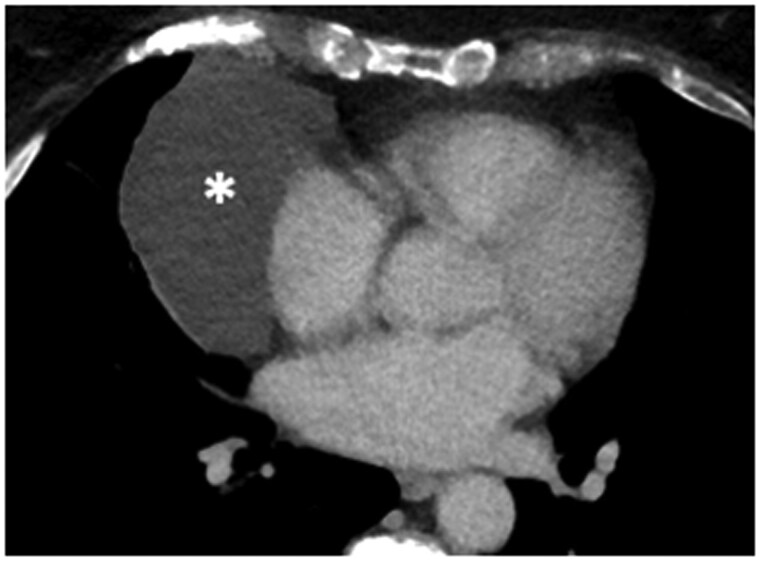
Pericardial cyst. Contrast-enhanced axial CT image of the chest reveals a lesion (asterisk) in the right cardiophrenic angle, characterized by fluid density and direct contiguity with the pericardial surface.
